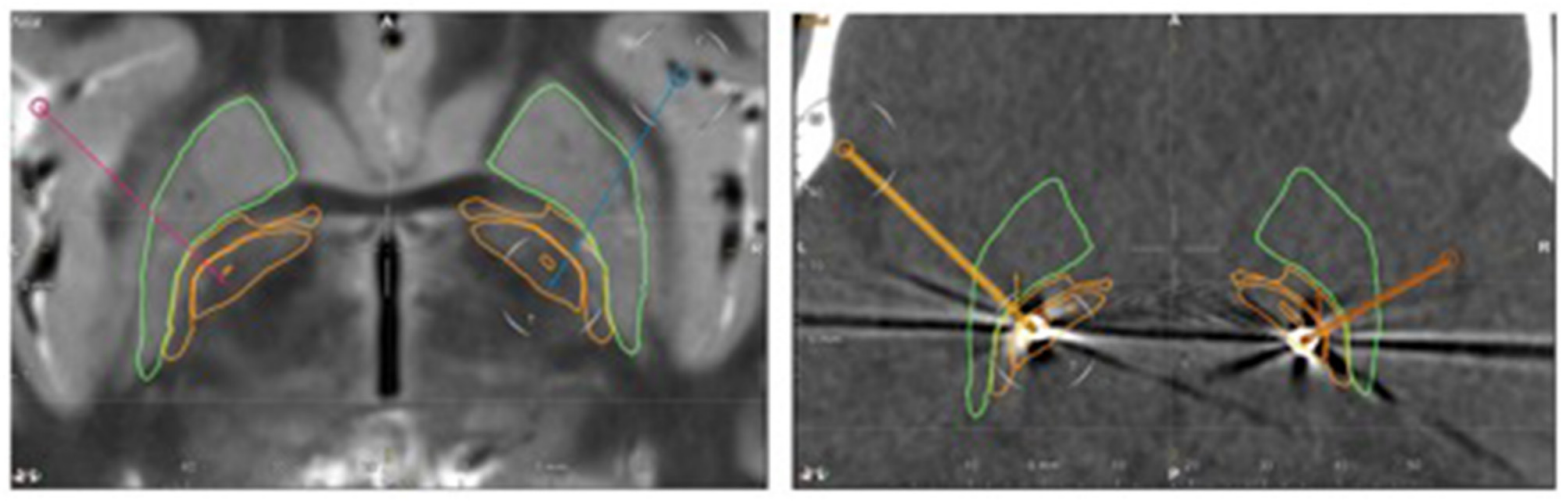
Left: Pre-operative axial T2-weighted MRI. Stereotactic planning performed with the Brainlab Elements software shows the planned trajectories (red and blue line). Right: postoperative axial CT. The pre-calculated electrode position is projected to the CT artifacts of the real electrode (yellow on the right side, orange on the left side). The leads are located within the caudal part of the GPi. Green outline: putamen, outer orange outline: globus pallidus externus, inner orange outline: globus pallidus internus.
